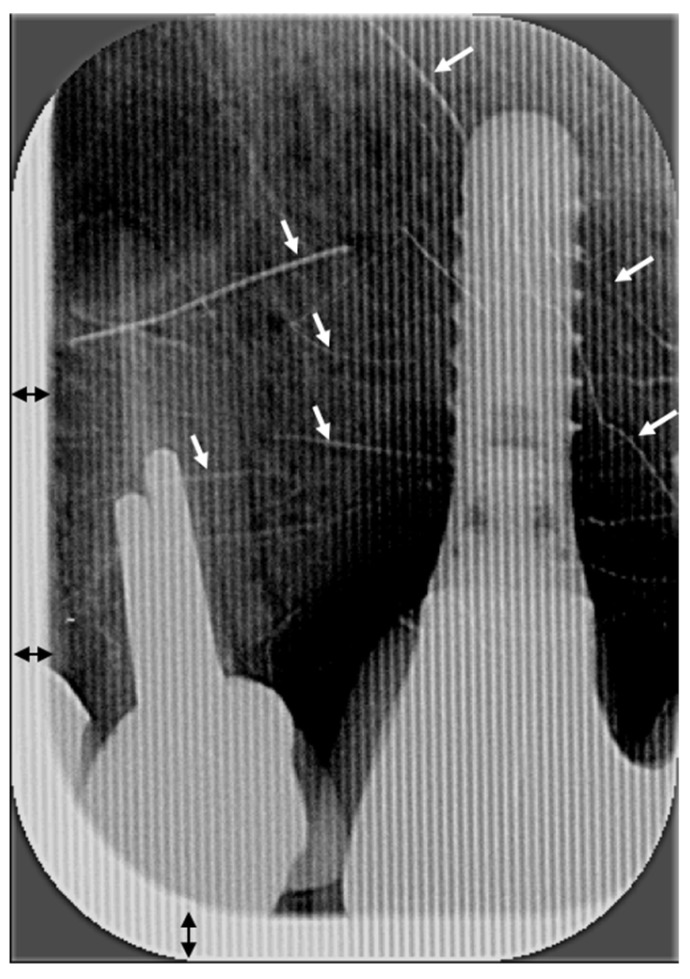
Vertical dark grey and white stripes superimposed on the dental implant, neighboring tooth, and dentoalveolar structures are due to malfunction of the digital plate scanner resulting in important loss of image quality. Multiple thin white scratches (shown with white arrows) in all directions are also visible. The truncated part of the X-ray, due to poor calibration of the photostimulable phosphor scanner, is shown with double black arrows.
